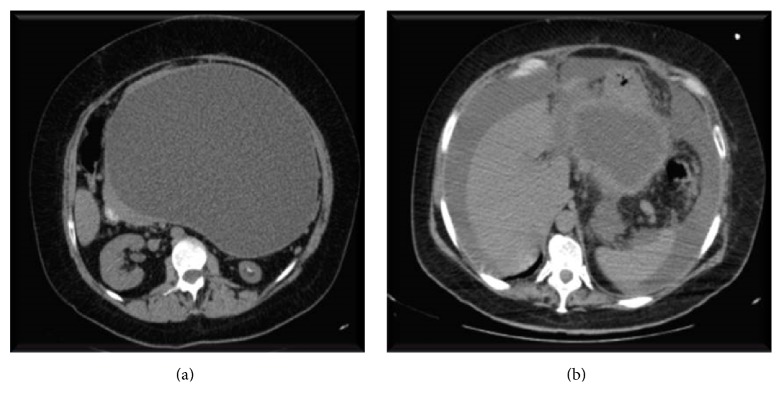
CT scan before and after pseudocyst rupture.
